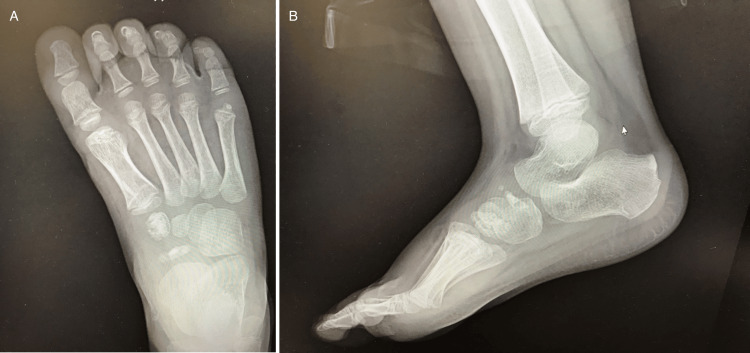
Radiographs obtained 10 weeks following initial diagnosis; anterior-posterior (A) and lateral (B) views of the right foot demonstrate progressive healing of the navicular and medial cuneiform with sclerosis and uniform osseous margins.
